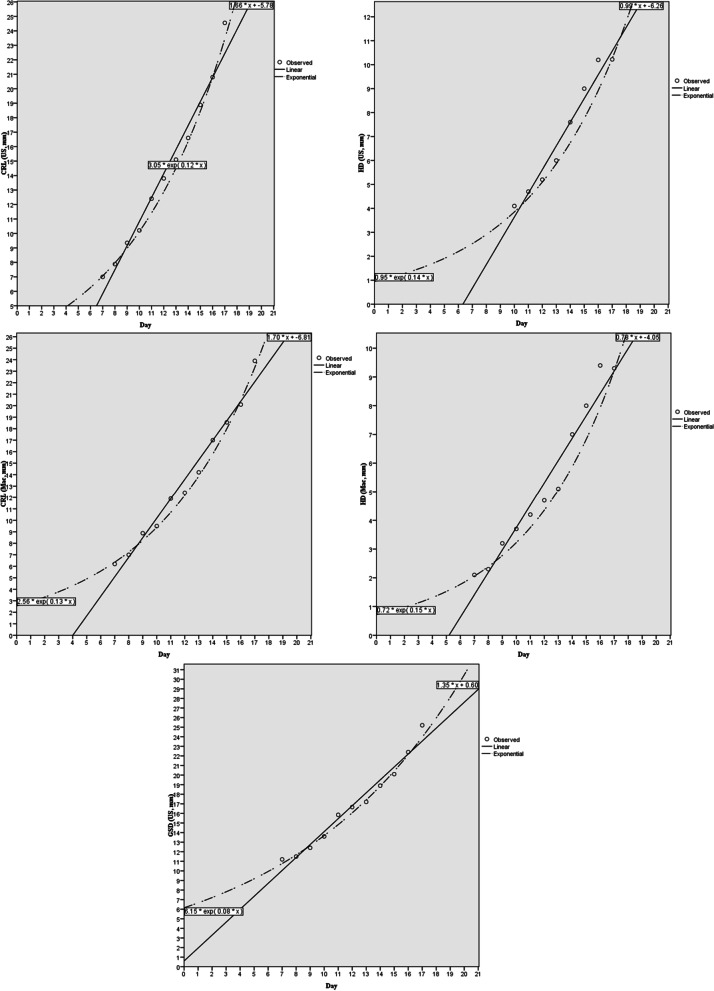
Correlation between days of pregnancy and ultrasonographic (us) and macroscopic (mac) measurements was presented for fetus and gestation sac of pregnant golden hamster. CRL: crown-rump length; HD: head diameter; GSD: gestation sac diameter
